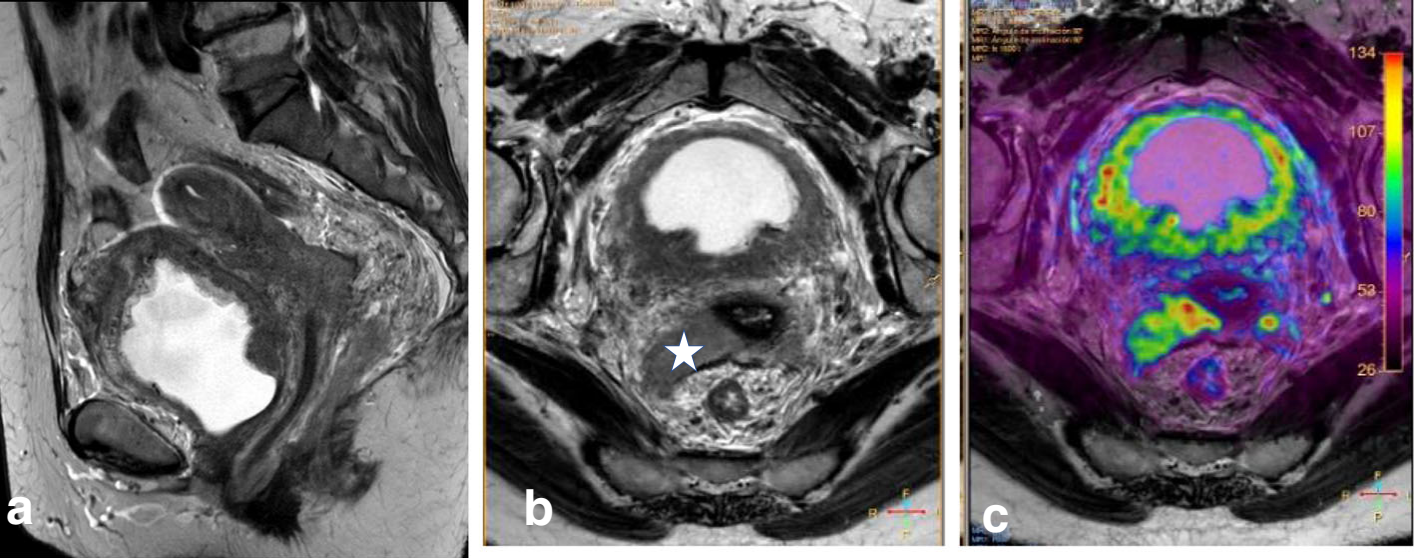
Transitional bladder cancer invading the cervix (star), visualized by sagittal T2WI (a), axial oblique T2WI (b), and T2WI+DWI (c)
